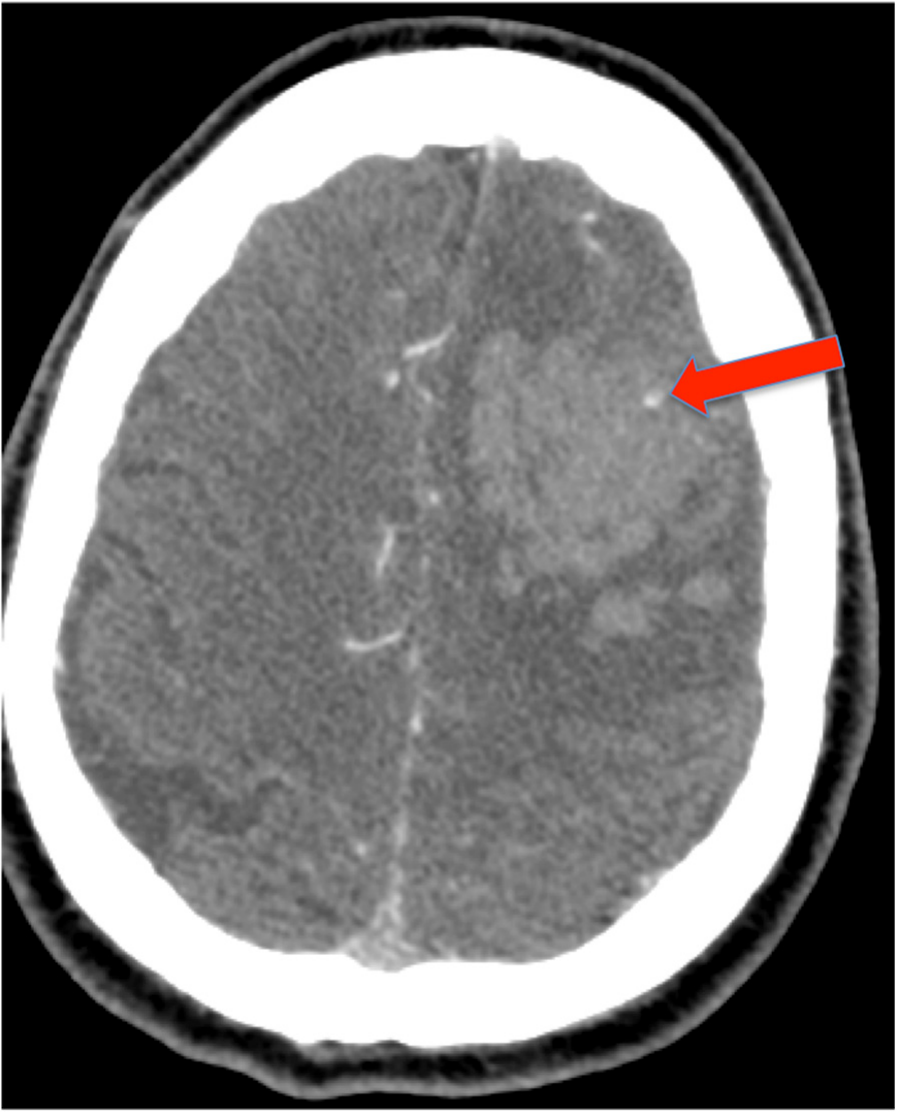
Spot sign. *Initially* described as contrast extravasation on CTA, the term has evolved to encompass foci of enhancement within the hematoma on CTA (*red arrow*)
